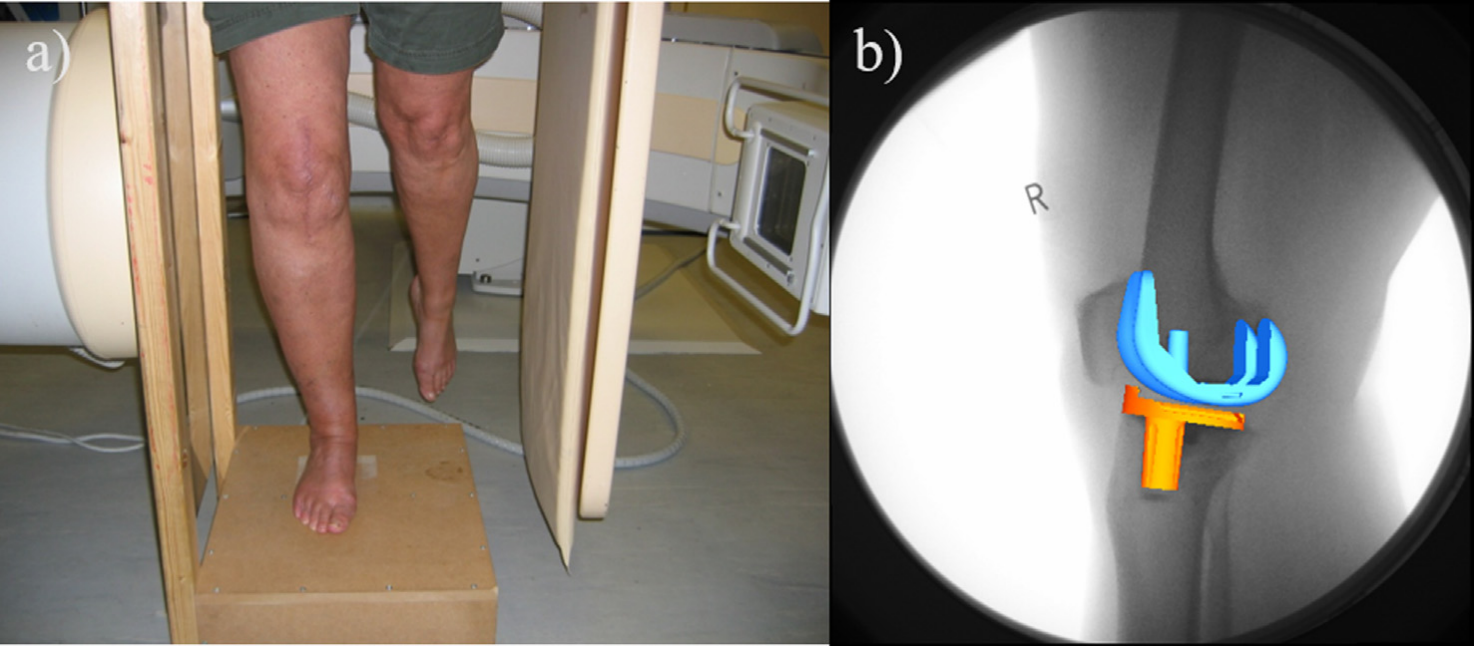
Fluoroscopy data collection and 3D to 2D image registration. a) Patient volunteer performing the step activity while video fluoroscopy is captured. b) Example processed fluoroscopy framed processed using JointTrack showing 3D computer models of the femoral and tibial component aligned with the 2D X-ray frame.
